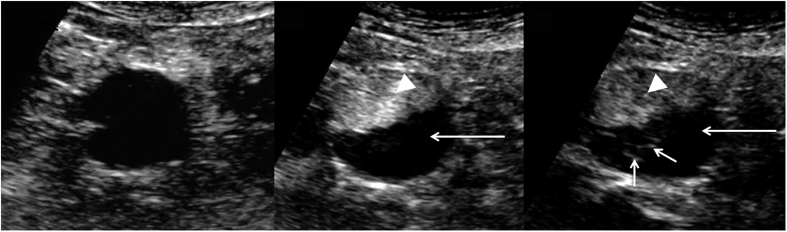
Transverse ultrasound examination of abdominal aortic aneurysm after the administration of contrast agent. (a) Ultrasound image at low mechanical index (MI) just before the contrast agent arrival; (b) images obtained 15 seconds after administration of the contrast agent, (b) images obtained 100 seconds after administration of the contrast agent. The hyperechoic channels within the hypoechoic thrombus are clearly visible (short arrows) (arrowhead - aortic lumen, long arrow-h hypoechoic thrombus).
